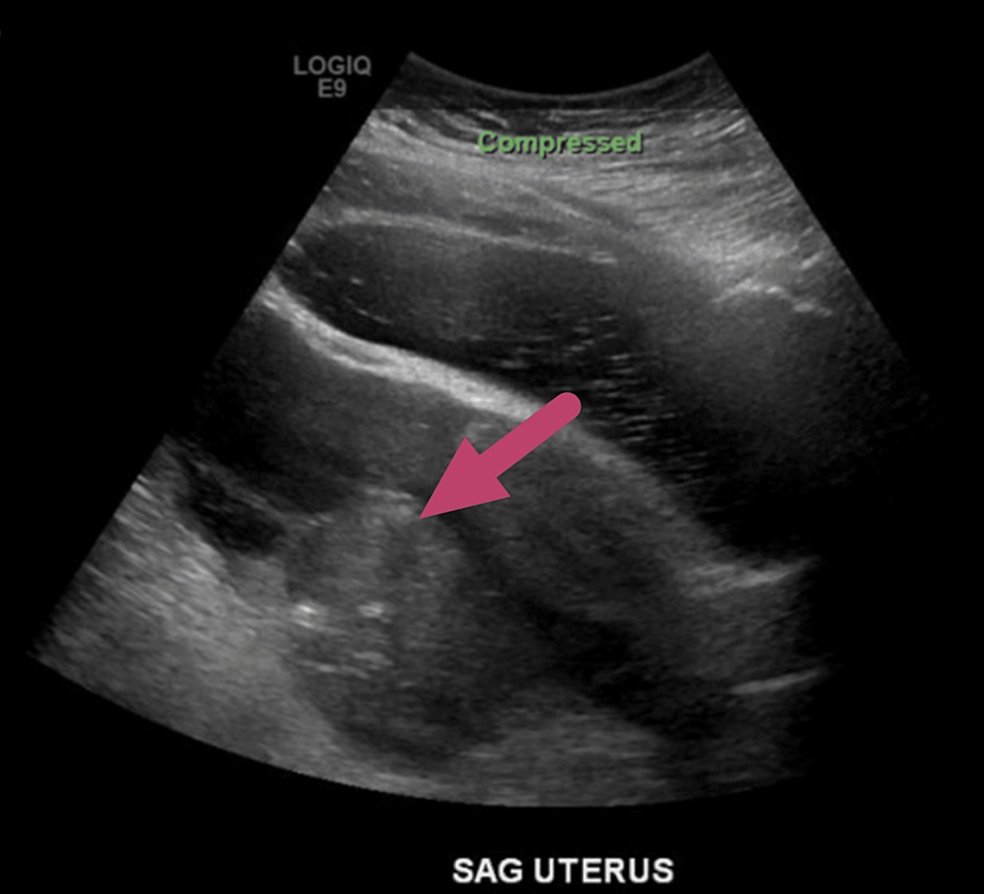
Ultrasound of the female pelvis and bladder showing a left adnexal mass (red arrow).
